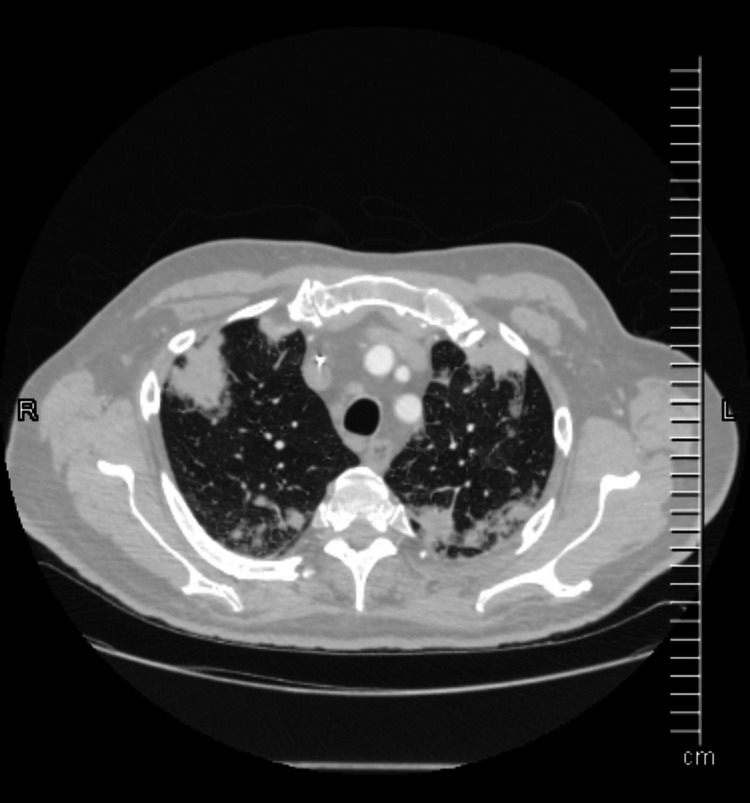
CT scan at presentation.
